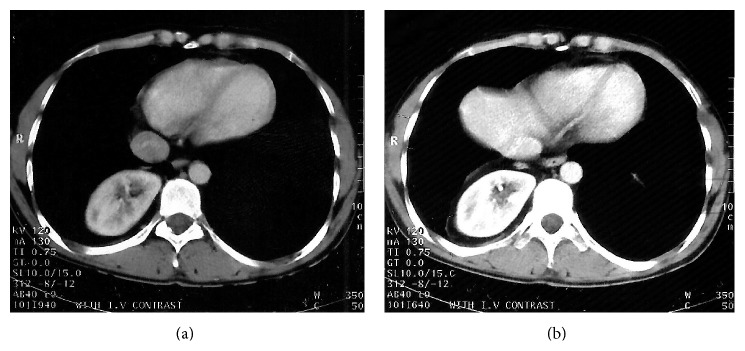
32-year-old man with thoracic ectopic kidney; an axial contrast enhanced CT scan of the revealed right kidney is in the lower portion of the right hemithorax.
